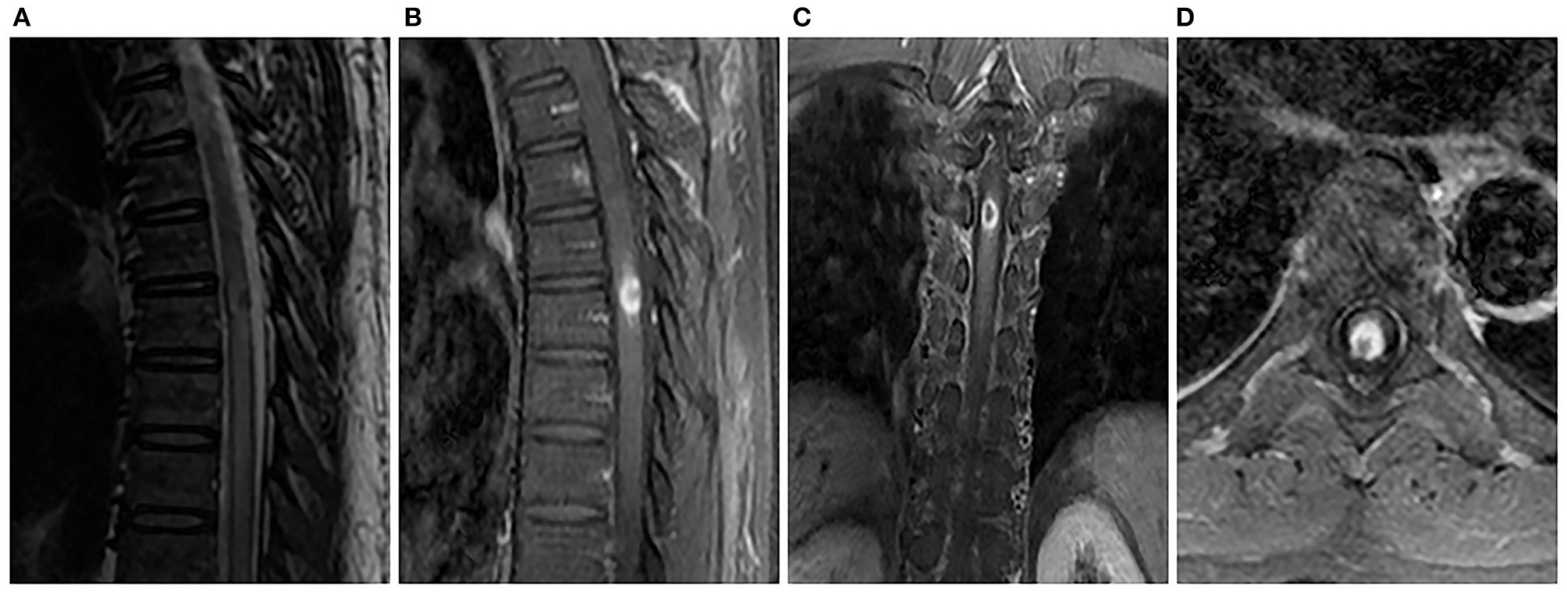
Spinal MRI showed an intramedullary heterogeneous nodule at the T5 level that was associated with extensive thoracic cord edema, while most hyperintense was associated with a marked hypointense core on sagittal T2-weighted image (A). Sagittal (B), coronal (C), and axial (D) T1-weighted images with contrast revealed obvious peripheral enhancement and no central enhancement.
